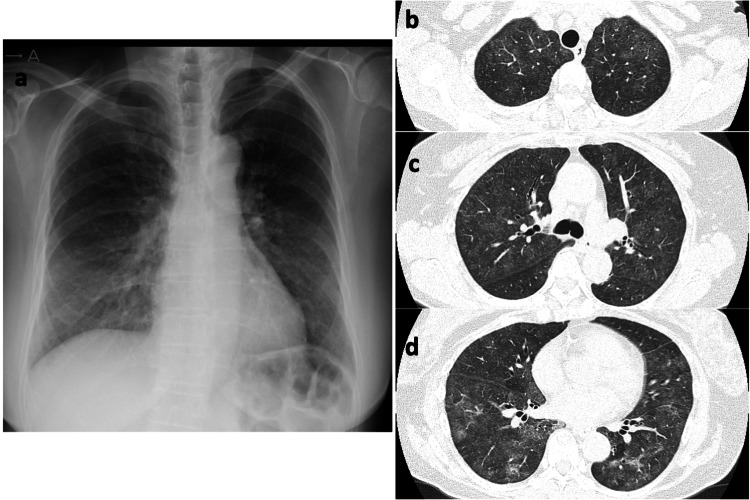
Chest X-ray and chest CT images of the patient. (a) Ground-glass opacities in bilateral lower lung fields on chest X-ray and (b-d) diffuse centrilobular ground-glass opacities with mosaic attenuation, along with areas of consolidation in the lower lobes of both lungs on chest CT
